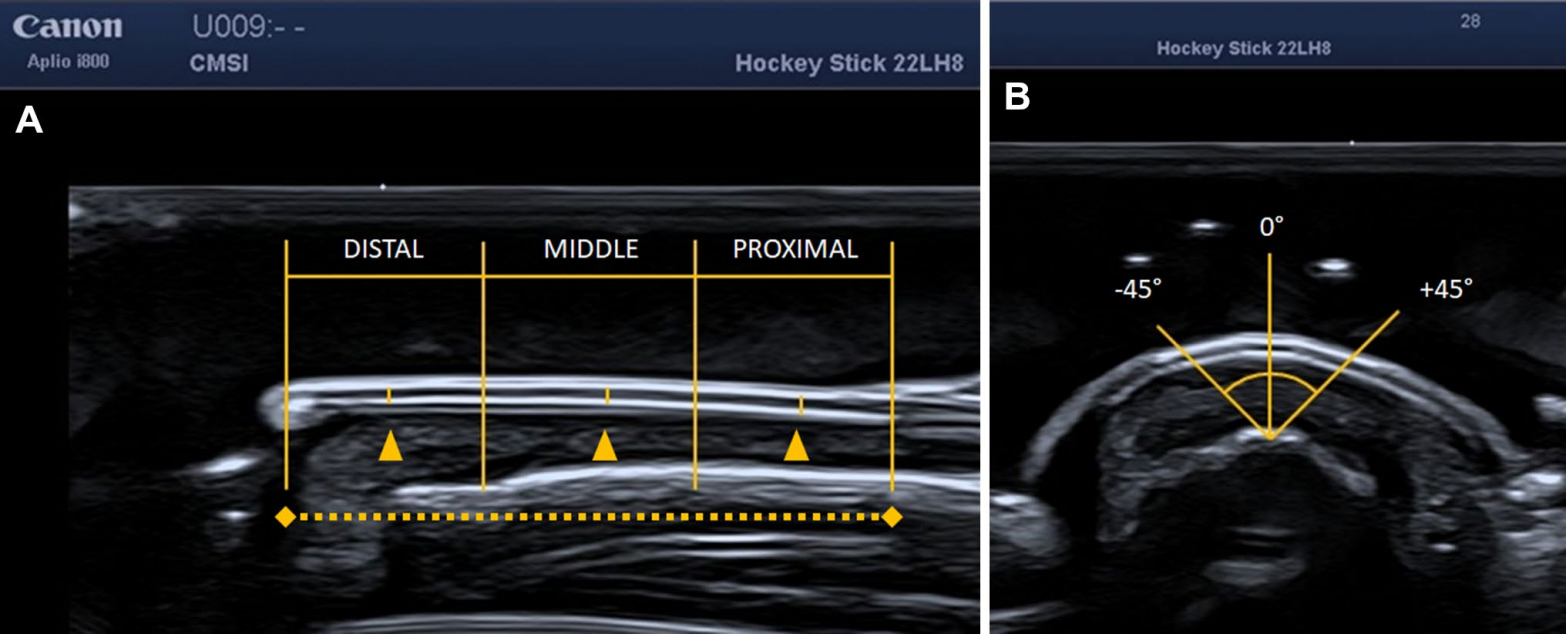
US appearance of the nail unit and points of reference for measurements. Below a consistent layer of gel (strongly hypoechoic), the first structure encountered was the sharp and hyperechoic band of the nail plate (dorsal lamina), then an anechoic part represents the intermediate lamina and the third lamina was the hyperechoic band (ventral layer of the nail plate). Then the nail bed appears as a hypoechoic space between the ventral layer of the nail plate and the hyperechoic cortical bone (area with arrowheads). The proximal longitudinal third stops at the eponychium. The nail matrix below the eponychium, a hypoechoic area with frequent Doppler signal is not shown in the figure. In picture A, the dotted line is the whole length of the nail considered for partition. This length was then divided in to three sections: distal, middle, and proximal. Every portion was then divided in half and at the middle the measurement of nail thickness was evaluated (arrowheads). In picture B, the transverse scan is marked by the angle tool of Weasis. Once the point of reference is obtained, the measurement was carried out carefully maintaining a 90° angle between the virtual ruler and the trilaminar structure of the plate
